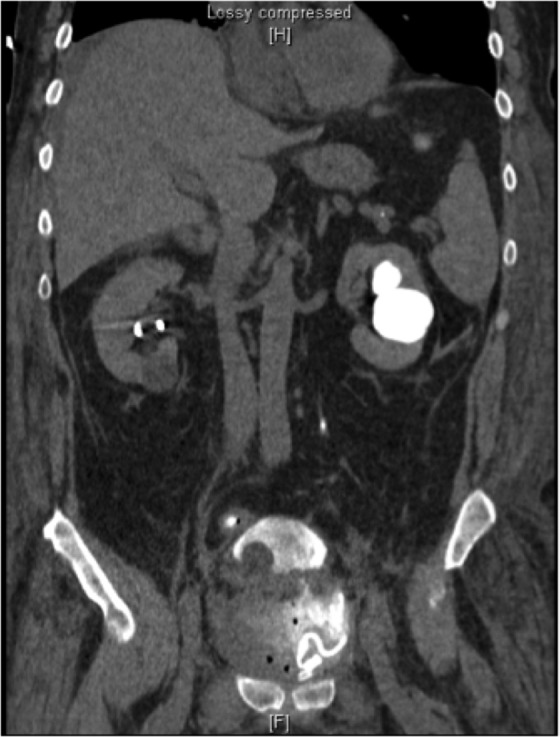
CT saggital abdomen/pelvis with contrast. Disruption of vesicourethral anastomosis with bladder displaced superiorly.
